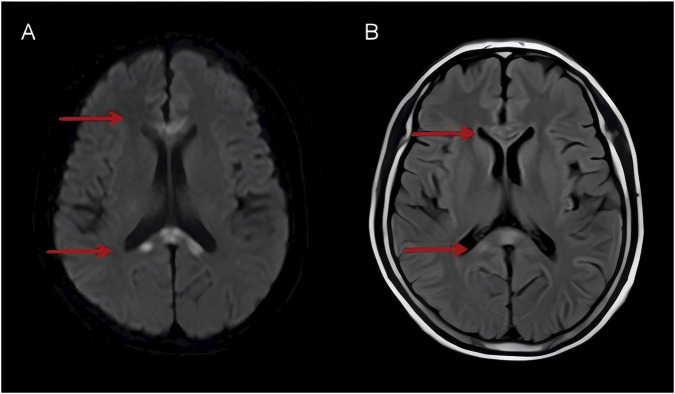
T2-FLAIR (A) and DWI (B) demonstrated improvement of the abnormal signals on DWI and FLAIR compared to prior images.
